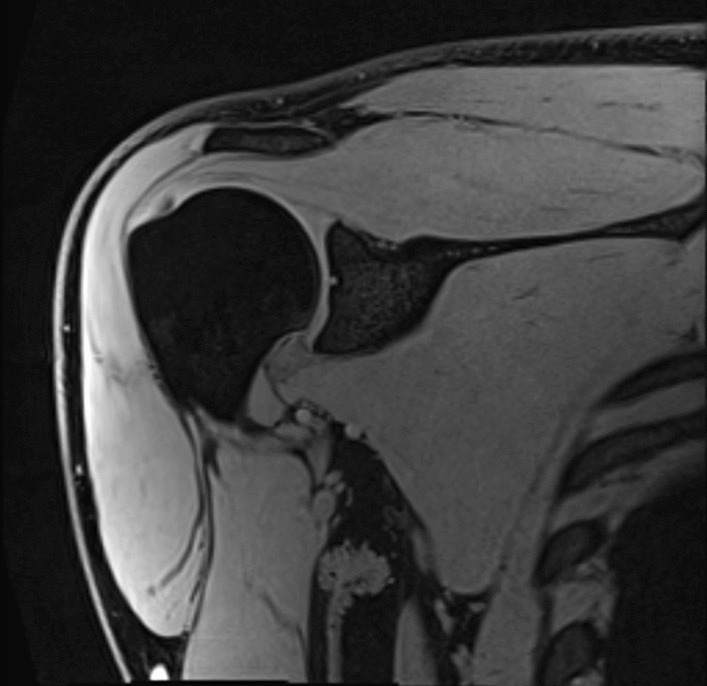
MRI image of the right shoulder using a T1 VIBE sequence in the coronal plane
